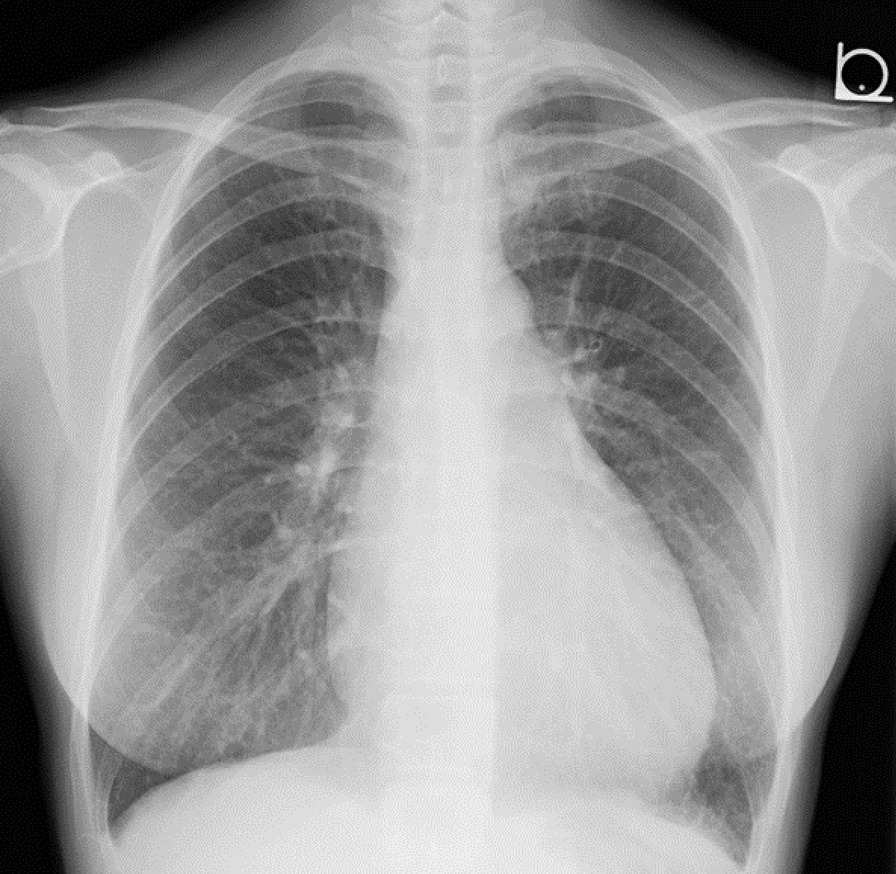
Chest radiograph acquired at the initial visit. The chest radiograph shows cardiomegaly. The cardiothoracic ratio is 52.4%
